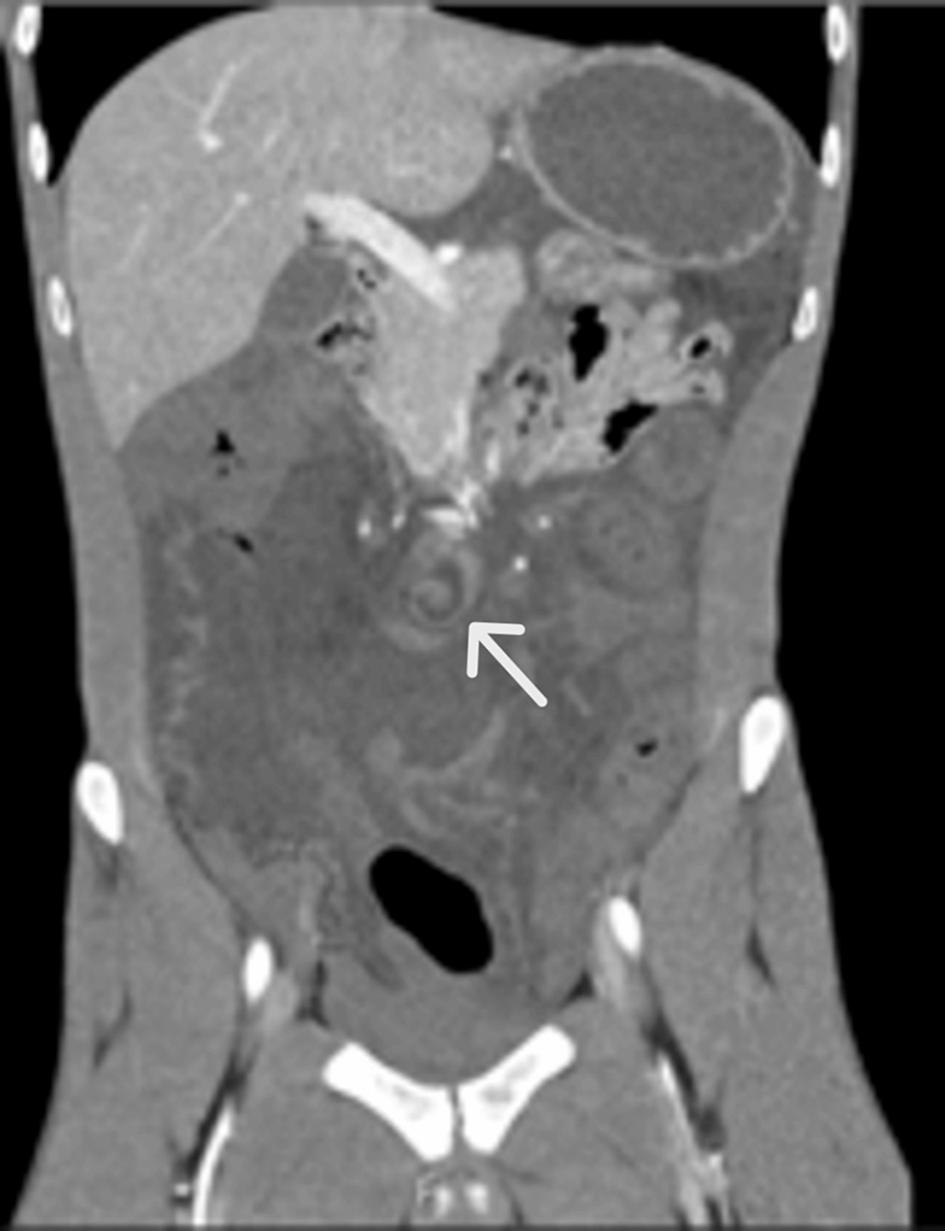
CT Scan coronal view: Whirlpool sign (arrow)
